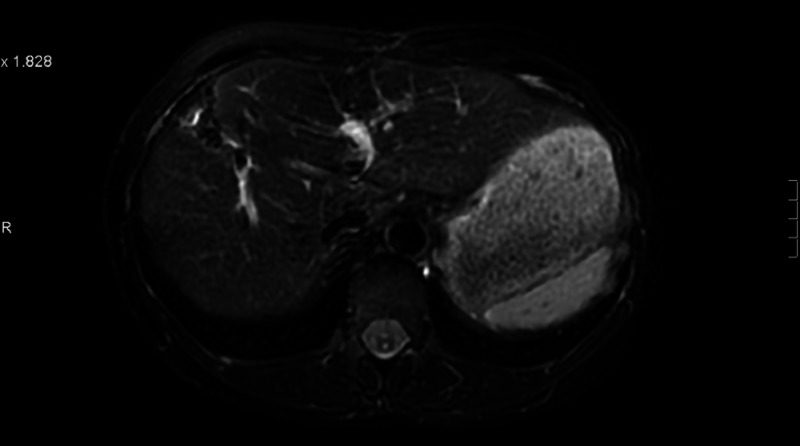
Patient 3: Magnetic resonance imaging (MRI) liver post resection demonstrates no evidence of recurrence
